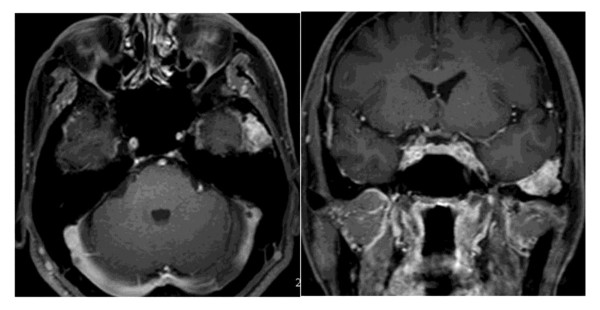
Axial and coronal magnetic resonance images with contrast show a left epidural mass at the middle cranial fossa.
